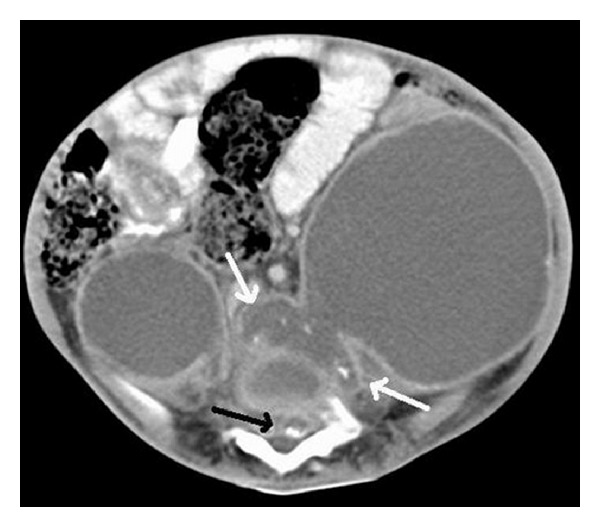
CT scan of abdomen and lumbar spine-axial section showing bilateral iliopsoas abscesses reaching up to the prevertebral region with a few hyperdense areas and bony fragments within (white arrows). Also seen is the epidural component in the spinal canal (black arrow).
